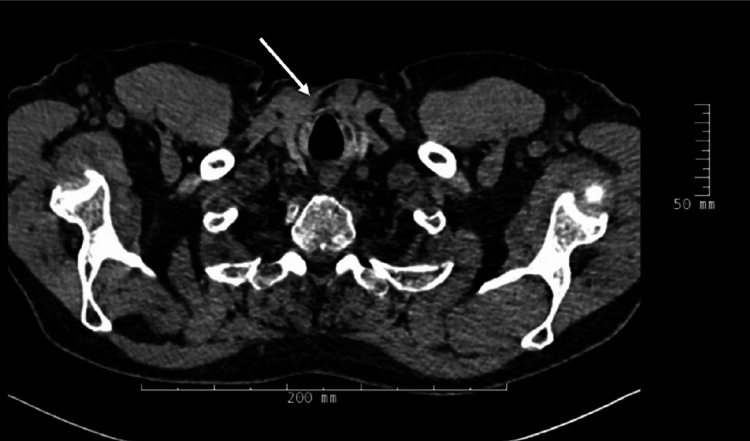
Chest CT without IV contrast after treatment
